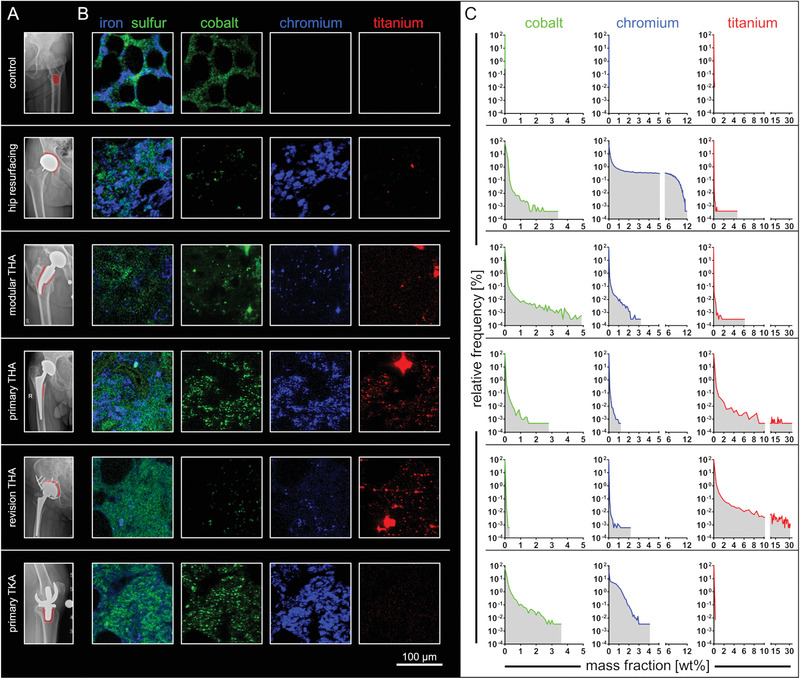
Co, Cr, and Ti were abundantly detected in peri‐implant bone marrow from various implant types and locations. A) Preoperative X‐ray radiographies. Red areas indicate the site of sample extraction. B) Qualitative RGB image sections of micro‐XRF maps with a resolution of 2 µm. Fe and S indicate matrix structures of the marrow. Mapping of Co, Cr, and Ti indicates the widespread dissemination of these metals in peri‐implant BM. Brightness and contrast of the RGB images were adjusted to represent the individual minimum and maximum amplitude of the signal. Scales are therefore not comparable from one sample to another. C) Frequency distributions of quantified mass fractions of each individual map and element indicate supraphysiological signals of Co, Cr, and Ti if compared to control (bin width [wt%]: Co, 0.1; Cr, 0.1; Ti, 0.5). Abbreviations: THA, total hip arthroplasty; TKA, total knee arthroplasty.
